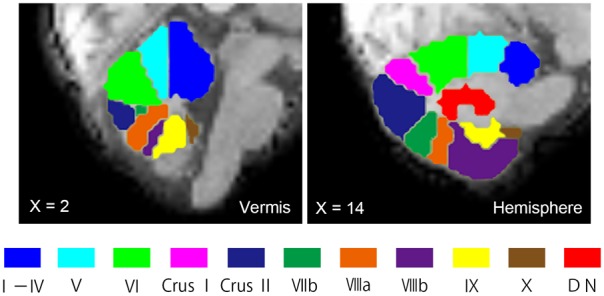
Seed ROIs in the cerebellum used for FC analysis. Seed ROIs for the vermis, the hemisphere, and the dentate nucleus of the cerebellum were created. The ROIs are overlaid onto sagittal images of the representative participant’s normalized T1-weighted images. An ROI for the vermis Crus I is not shown in this figure, as it does not appear in this vermal slice (*X* = 2). ROIs, regions of interest; FC, functional connectivity; DN, dentate nucleus.
